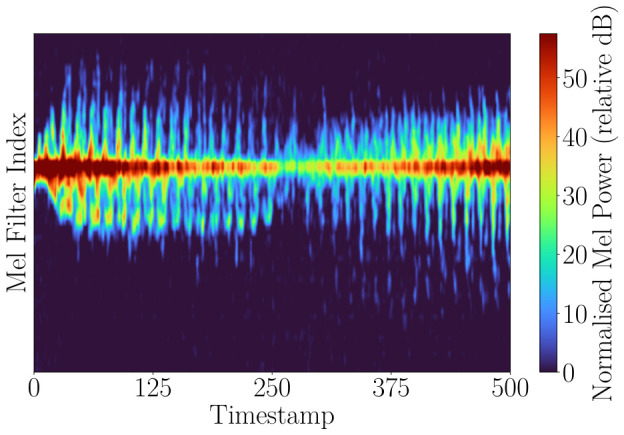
Block mel spectrogram corresponding to the detected region of interest for a walking human. The region is centered on the dominant Doppler component, reducing background interference and enhancing the visibility of limb-induced micro-Doppler variations compared to the global representation in Figure 5.
